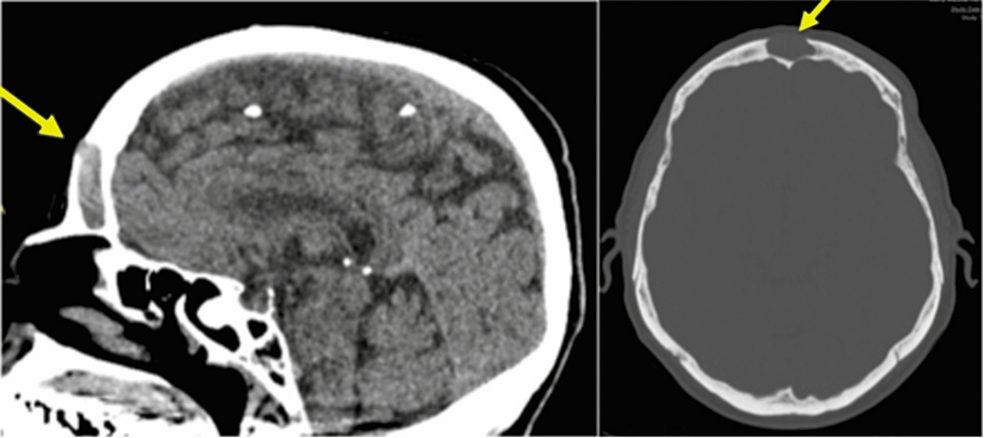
Head CT without contrast There was an expansion of the frontal sinus in the midline with loss of the anterior cortex (arrows). The maxillary sinuses and frontal sinuses were completely opacified with the walls of the maxillary sinuses being sclerotic. The orbits were normal, and the mastoid air cells were clear. There was no intracranial hemorrhage, mass, or CT evidence of infarct.
